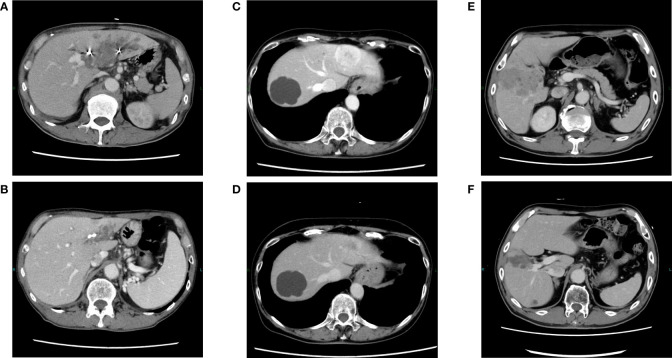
Changes in contrast-enhanced CT findings in responders during treatment. Pretreatment with atezolizumab plus bevacizumab in cases 1 (A), 2 (C), and 5 (E). After treatment with atezolizumab plus bevacizumab in cases 1 (B), 2 (D), and 5 (F), in which a partial response was observed.
